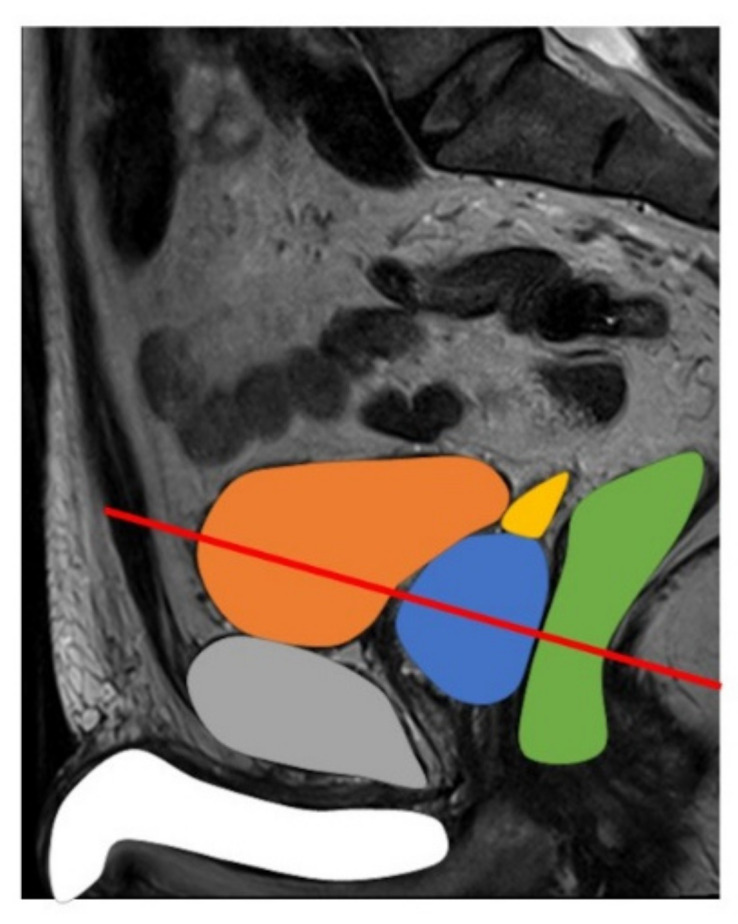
Sagittal view of male pelvis on MRI: bladder–orange, prostate–blue, seminal vesicles–yellow, rectum–green, pubic bone–grey, penis–white. Red line indicates plane of image with the endorectal coil located in the rectum.
